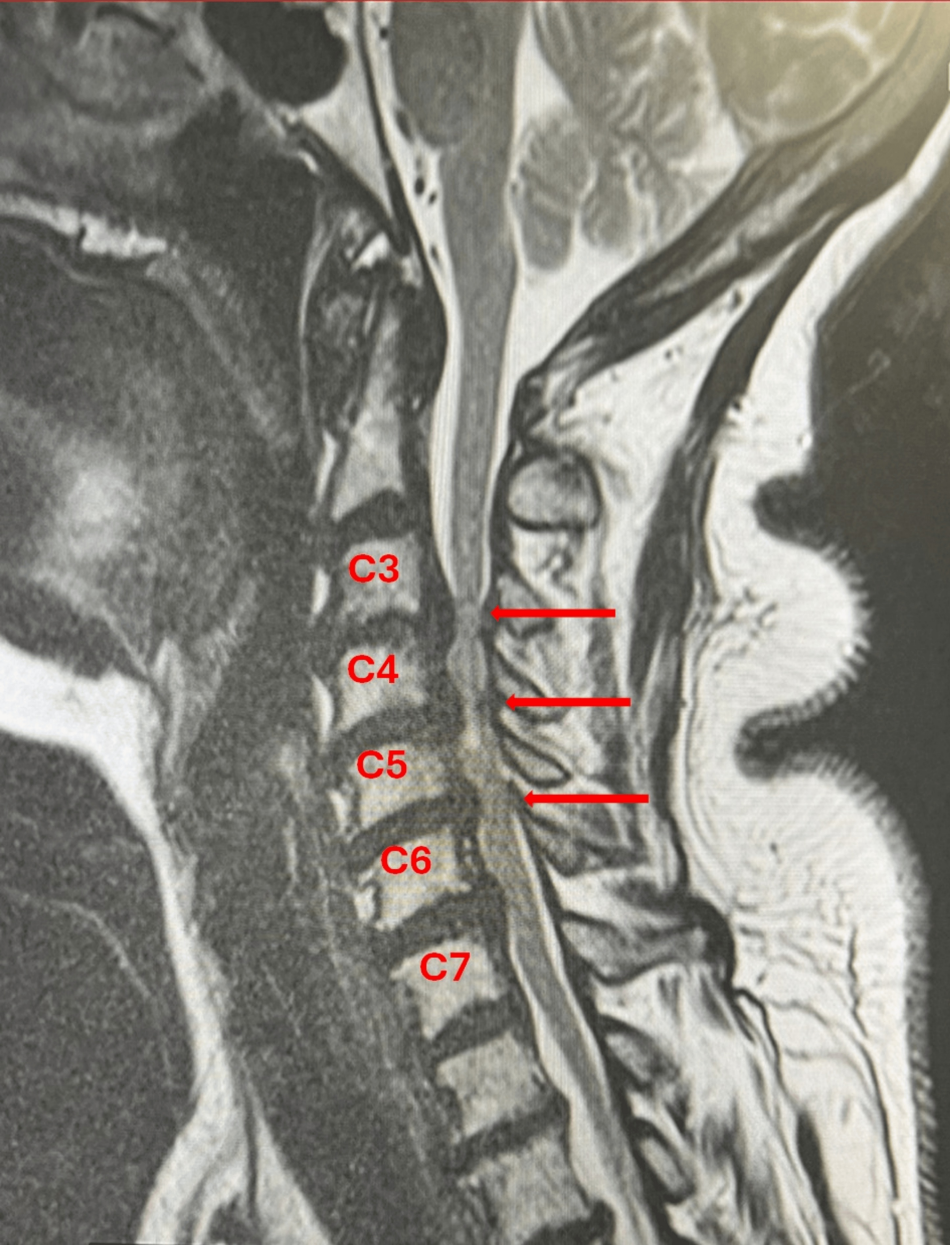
Preoperative cervical spine sagittal T2-weighted MRI. Red arrows show multilevel cervical stenosis.
